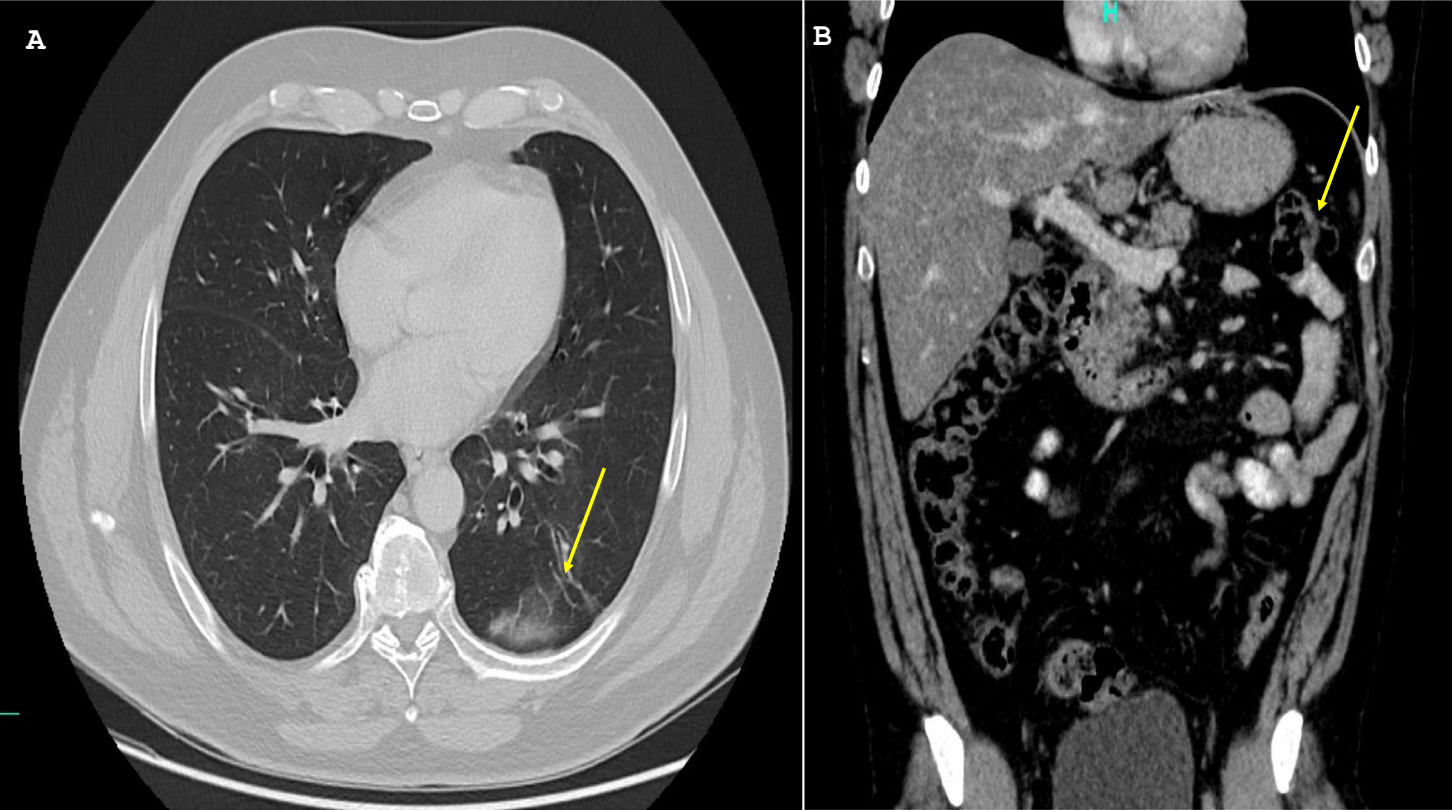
Follow-up contrast-enhanced computed tomography images obtained in 2020 demonstrating a complete radiologic response. A Axial chest computed tomography showing complete radiologic response of the previously identified pulmonary metastasis (arrow). B Axial abdominal computed tomography demonstrating complete radiologic response of the previously described abdominal metastatic lesions (arrow)
